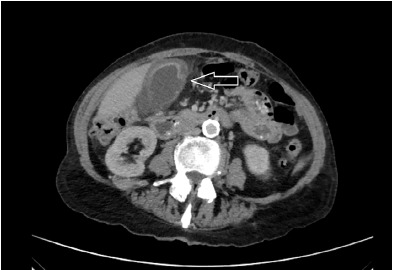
Abdominal computed tomography scan with contrast showing cholecystitis with evidence of gallbladder wall perforation (white arrow). Gallbladder wall appears thickened and edematous and there is a moderate amount of pericholecystic fluid and inflammatory stranding. In addition, there is an apparent discontinuity in the gallbladder wall.
